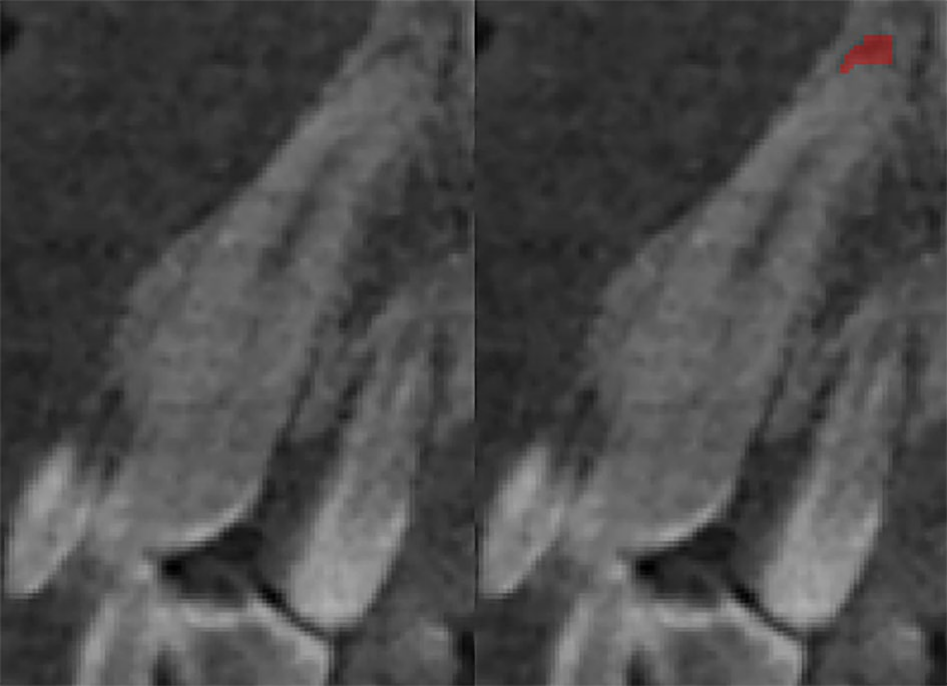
Periapical lesion localization mask at tooth 13.
